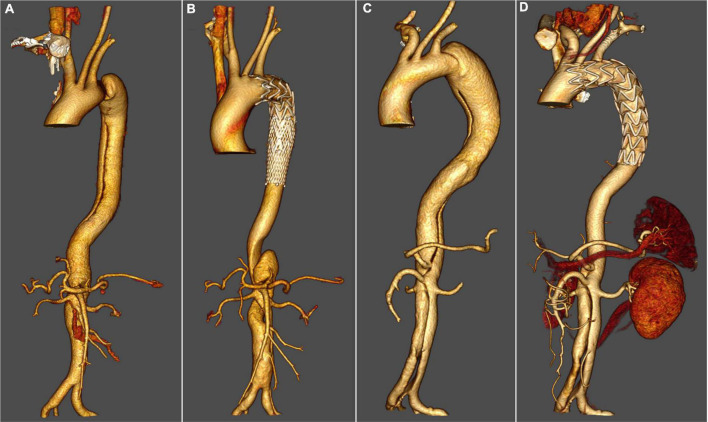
Thoracic endovascular aortic repair with fenestrated surgeon-modified stent graft. (A) Pre-operative three-dimensional computed tomography angiography (CTA) reconstruction for a patient with zone 1 TEVAR. (B) Three-dimensional CTA reconstruction for a patient with zone 1 TEVAR 3 years after the operation. (C) Pre-operative three-dimensional CTA reconstruction for a patient with zone 2 TEVAR. (D) Three-dimensional CTA reconstruction for a patient with zone 2 TEVAR 4 years after the operation.
